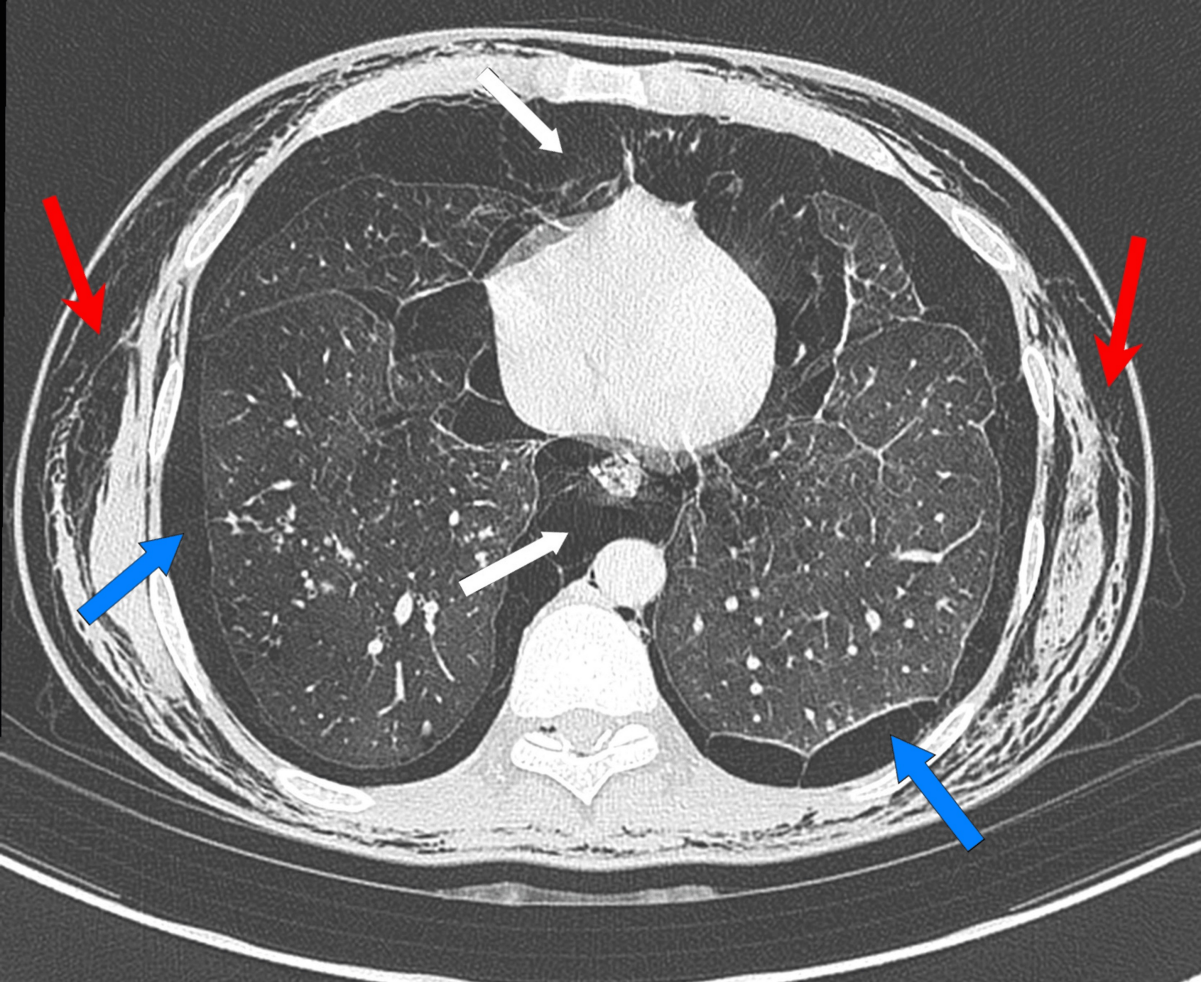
CECT chest scan of the chest revealed extensive fibrocavitatory disease, bilateral pneumothorax, pneumomediastinum, and widespread subcutaneous emphysema. CECT: contrast-enhanced computed tomography Red Arrows: Subcutaneous Emphysema; Blue Arrows: Bilateral Pneumothorax; White Arrows: Pneumomediastinum
